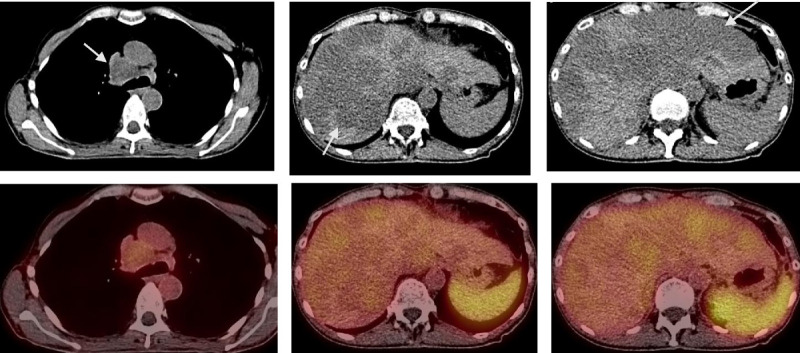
Major change: chemotherapy or biologic therapy. Computed tomography and fused ^68^Ga-DOTATOC PET-CT images through mediastinal nodes (arrow in left column) and through the liver (arrows in mid and right columns). Neither large mediastinal node nor hypodense liver lesions show uptake of ^68^Ga-DOTATOC. Patient is not considered a candidate for PRRT.
